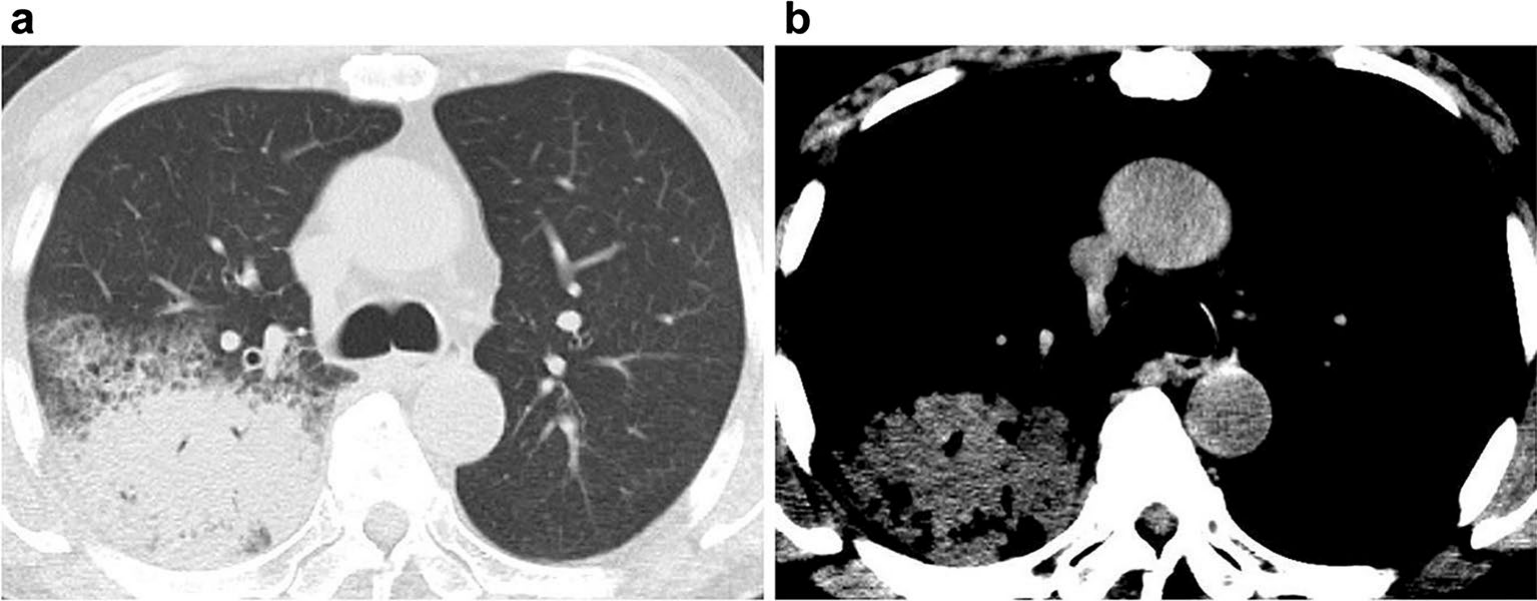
77-year-old man with bacterial pneumonia. (a) Transaxial CT with a lung window setting showing consolidations in the right lung. (b) In the narrow window setting with a mediastinal filter, the consolidation is iso- to slightly hypodense relative to the density in the aorta, indicating that the findings are negative for the hyperdense consolidation sign.
